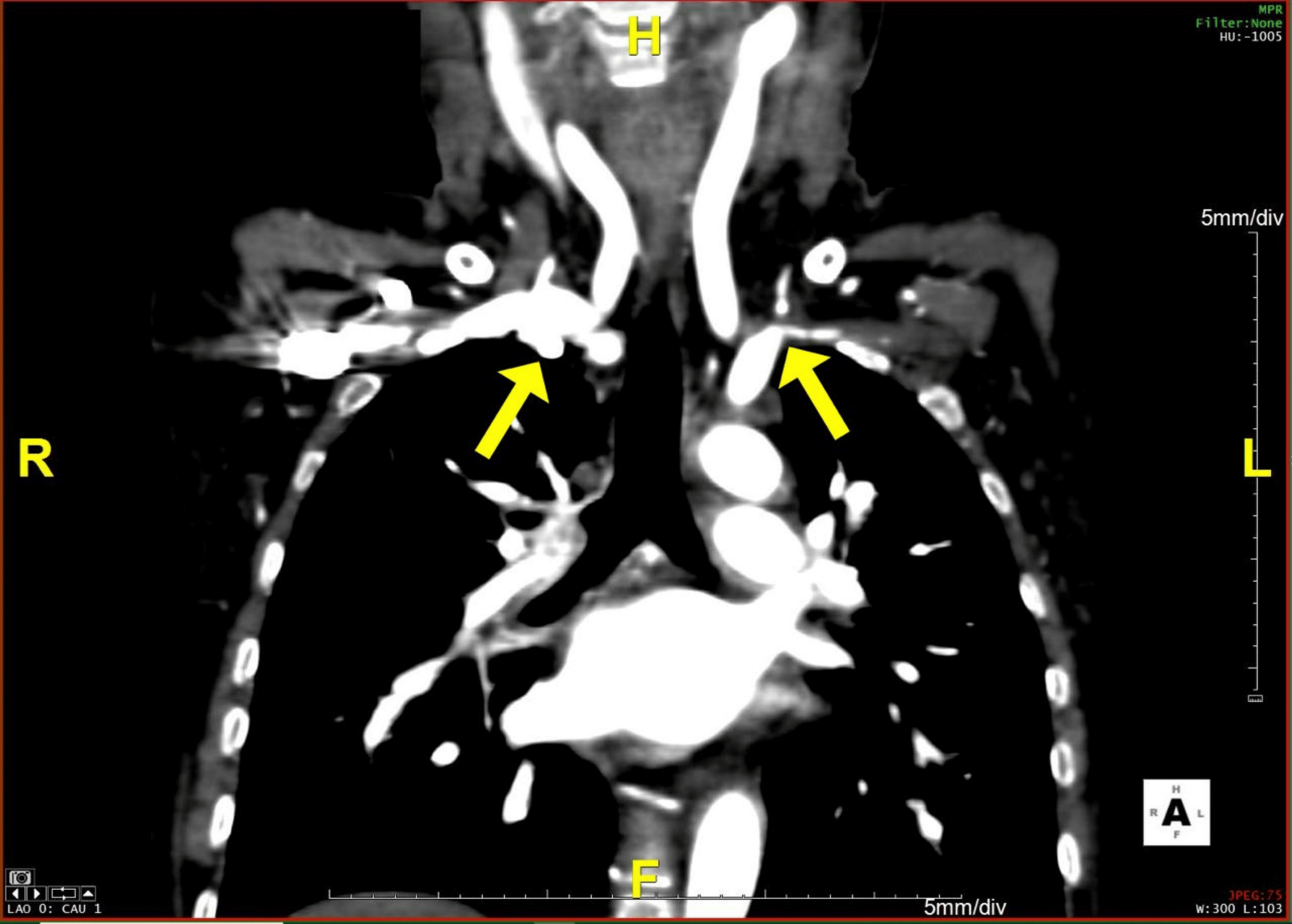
A coronal section of the CT angiogram comparing both subclavian arteries The image shows stenosis in the left subclavian artery CT: computed tomography
